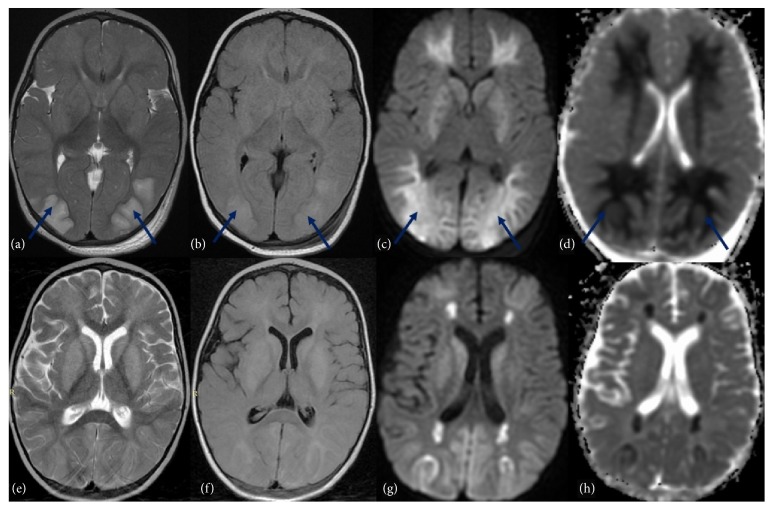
MRI scans: (a, e) T2-weighted; (b,f) Fluid Attenuated Inversion Recovery (FLAIR); (c,g) diffusion-weighted imaging; (d,h) apparent diffusion coefficient (ADC) map. The first MRI imaging (a,b,c,d) showed bilateral and symmetrical hyperintensity (
